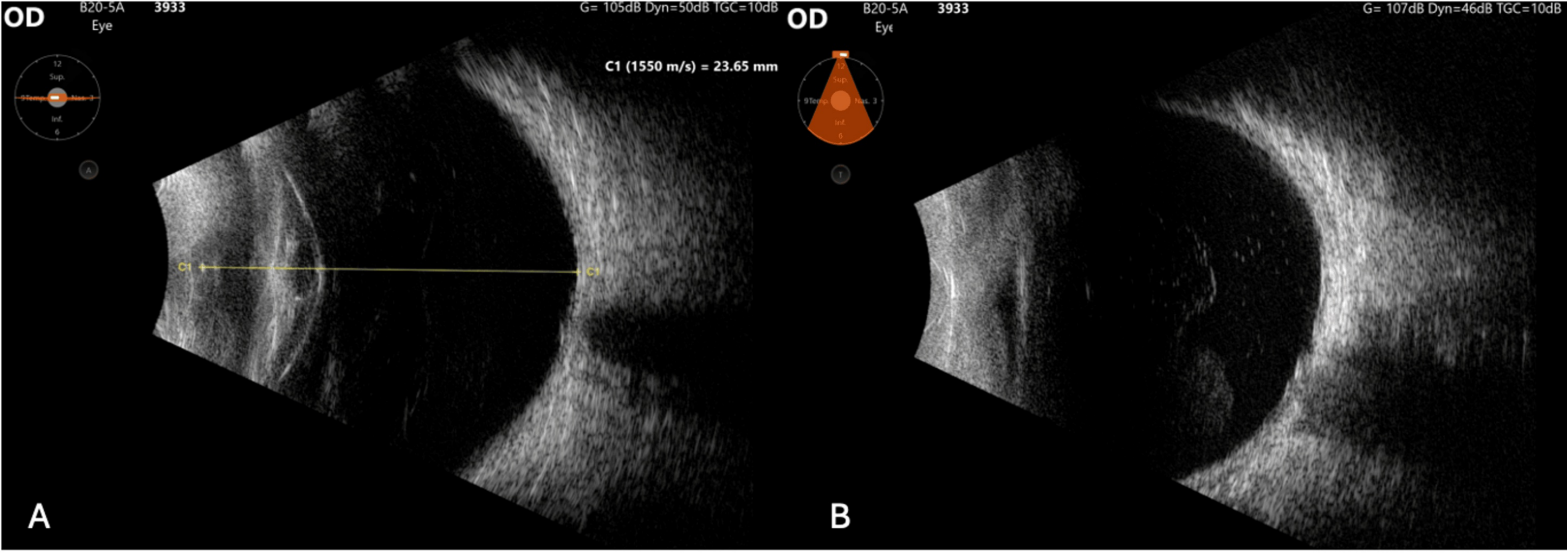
B-mode ultrasound of the right eye (A) Axial and (B) inferior transverse views, showing a dense cataract with fine and mobile punctiform echoes in the vitreous cavity
